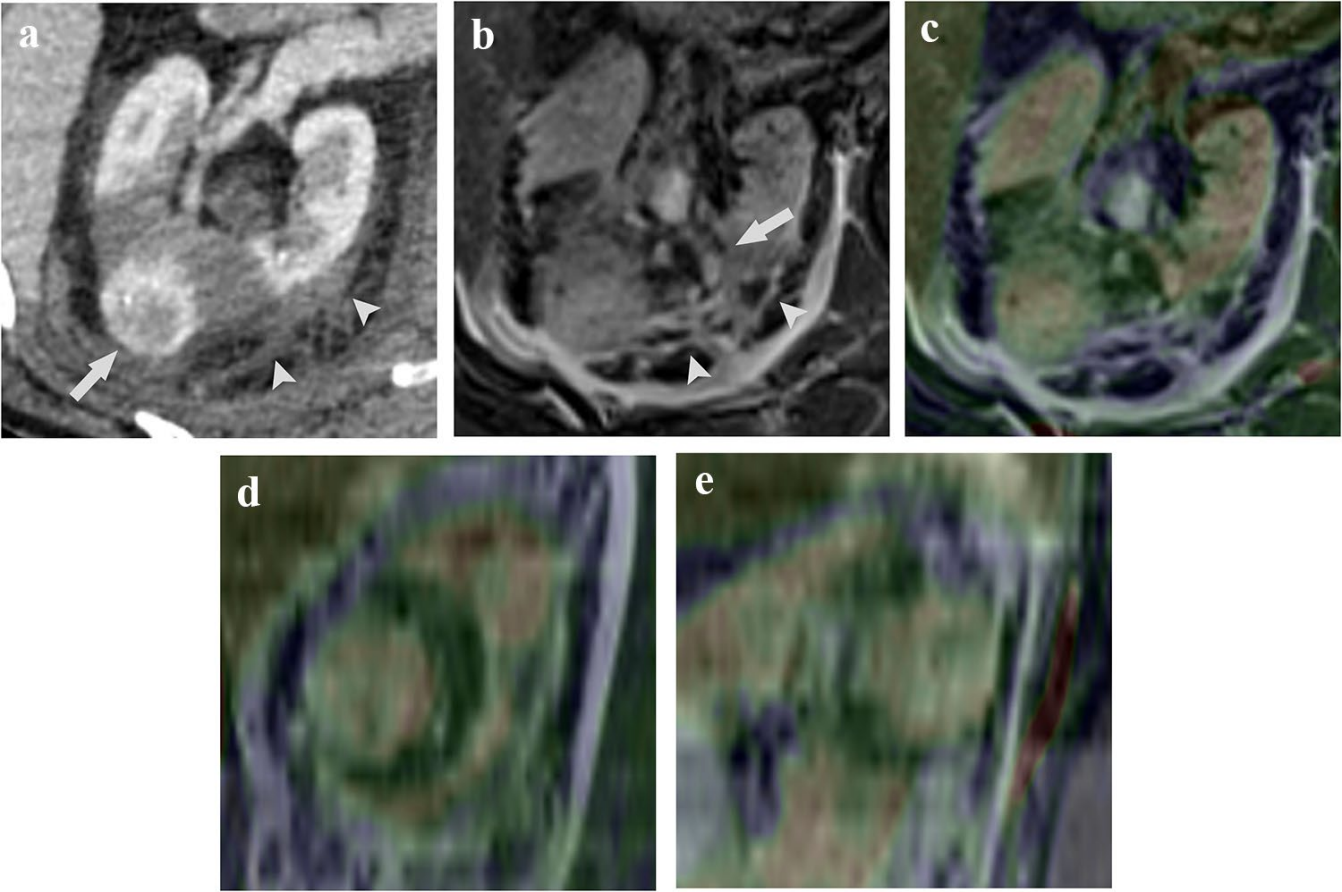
86 year-old man with biopsy-proven clear-cell renal cell carcinoma with a maximum diameters of 2.6 cm who underwent percutaneous cryoablation the day before. A In the nephrogenic phase of dynamic CECT image, the ablation zone is visualized as the unenhanced region with a strong appearance of perinephric changes, such as fluid collection and hematoma (arrowhead). Residual heterogeneous tumor enhancement (arrow) is shown. B In the fat-suppressed T2-weighted MRI image, perinephric changes such as fluid collection and hematoma show heterogeneous signal intensity changes (arrowhead), and the outline of the kidney is unclear. Although the therapeutic region shows hypointensity relative to the renal parenchyma, the left border with the renal parenchyma is slightly unclear (arrow). In this case, the renal volume of the regions with signal intensity changes on non-enhanced MRI was calculated to be 9.39 cm^3^ larger than the non-enhanced regions on CECT. The minimal margin on MRI was 4 mm. C, D, E, Fusion images combining the fat-suppressed T2-weighted MRI image and the nephrogenic phase of dynamic CECT image. Axial (C), coronal (D), and sagittal (E) images of the maximum cross-section of the tumor. The average match score of the two board-certified diagnostic radiologists on a 5-point scale is 4.3 points (radiologist 1 [axial, 4; coronal, 4; sagittal, 4] and radiologist 2 [axial, 4; coronal, 5; sagittal, 5])
